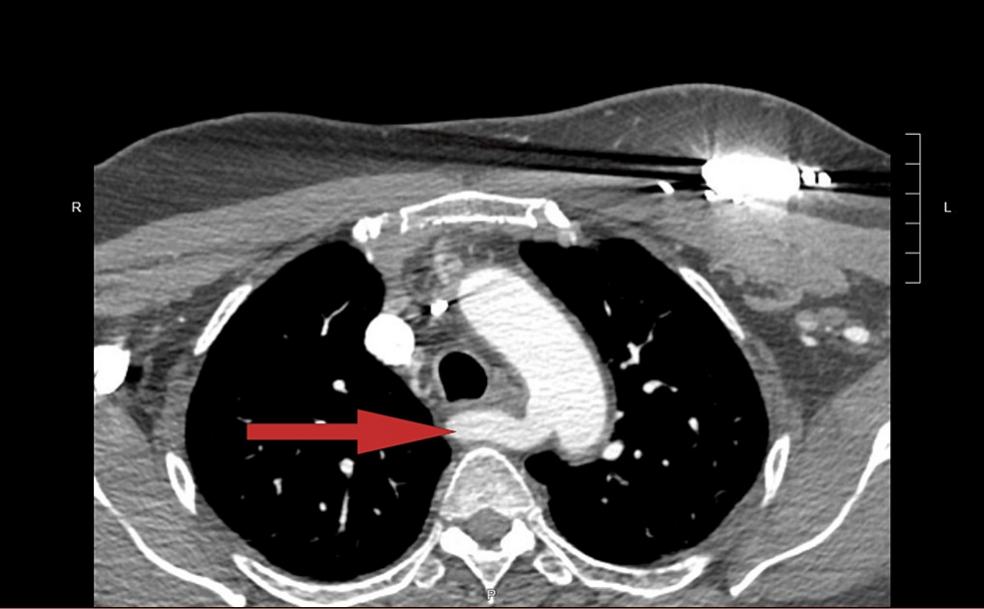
The chest CT angiography image displays an aberrant right subclavian artery (indicated by the arrow) originating from the aortic arch.
